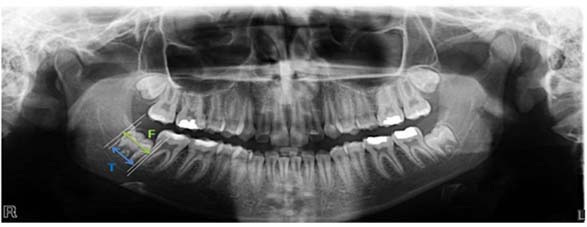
Radiographic measurement for the F/T ratio: follicle diameter (F) mesiodistal width of impacted tooth crown (T).
